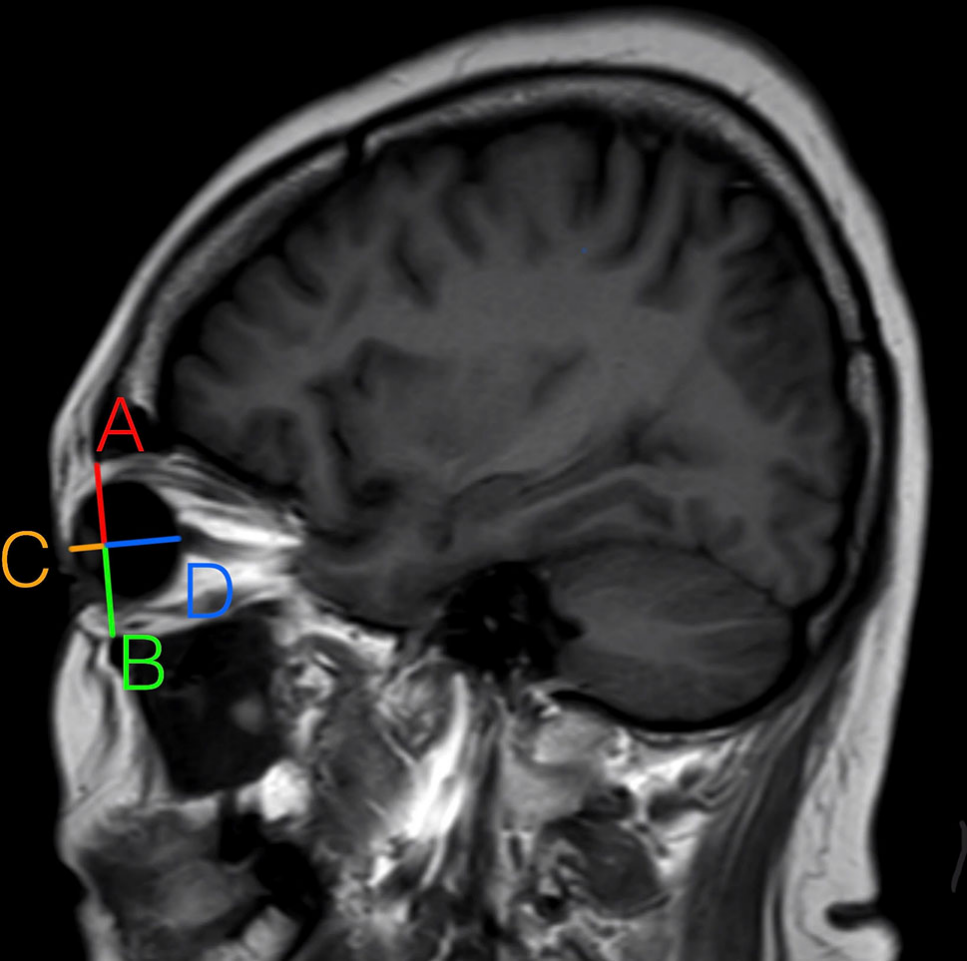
Sagittal view of MRI and globe position in anteroposterior and vertical positions
